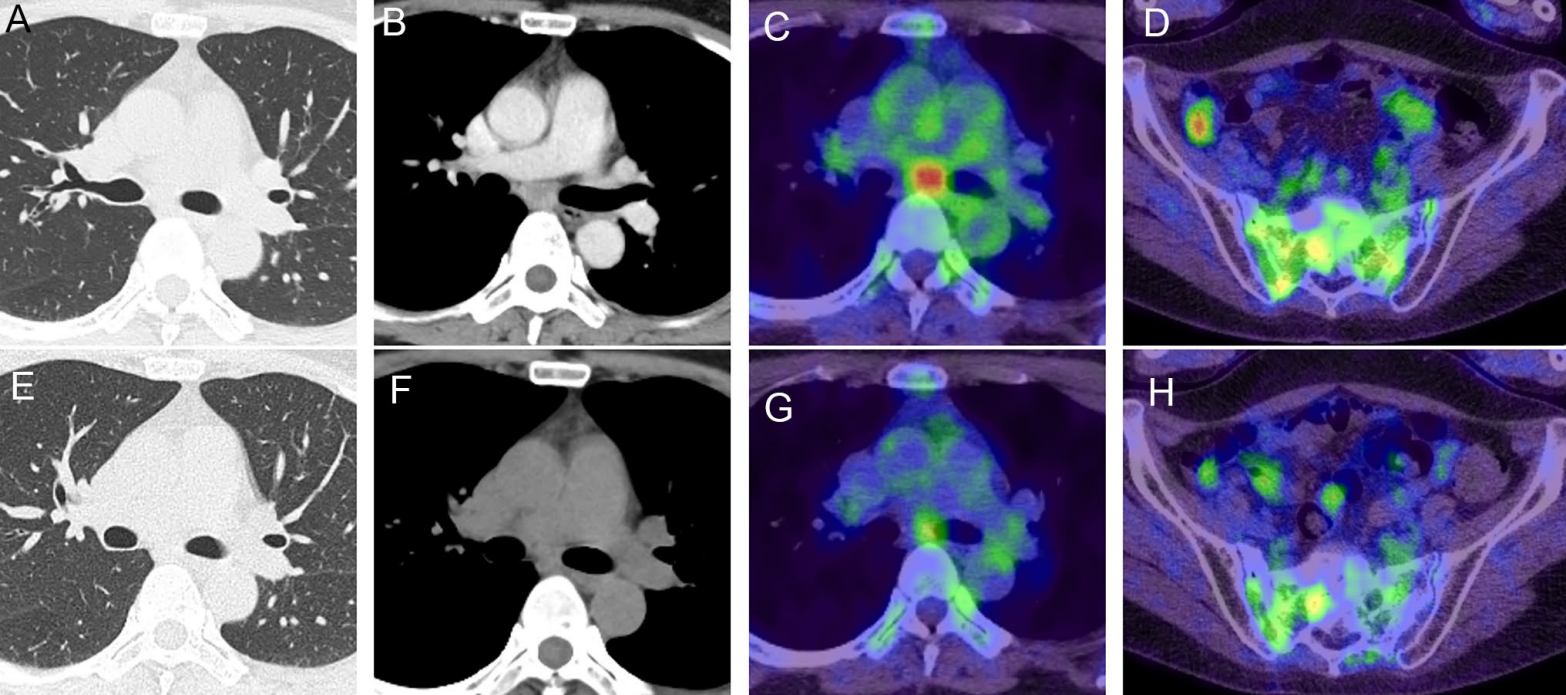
A, B, E, and F Chest CT. C, D, G, H PET-CT. A–D reveal enlarged mediastinal lymph nodes and FDG uptake in the lymph nodes and sacrum, indicating recurrent PSP. E–F Images obtained after three immunotherapy cycles revealing reduced mediastinal lymph node size and FDG accumulation. *CT* computed tomography, *PET-CT*, positron emission tomography-computed tomography, *FDG* fluorodeoxyglucose, *PSP*, pulmonary sclerosing pneumocytoma
